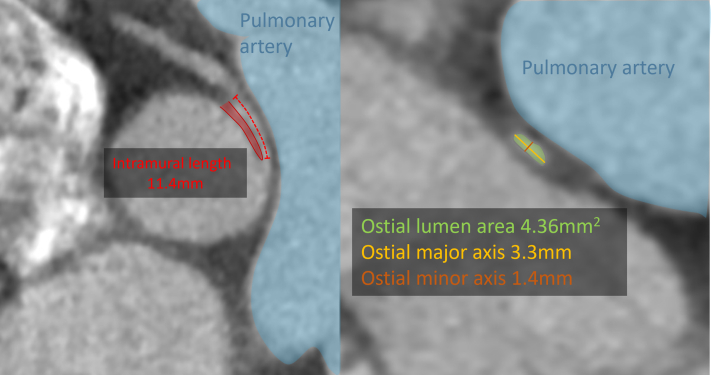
CCTA Images (Left) Intramural course length of 11.4 mm, with the interluminal space between the aorta and the coronary artery highlighted in red and the pulmonary artery in blue. (Right) Ostial lumen area of 4.36 mm^2^, with a major axis of 3.3 mm and a minor axis of 1.4 mm. The pulmonary artery is again shown in blue. CCTA = coronary computed tomography angiography.
